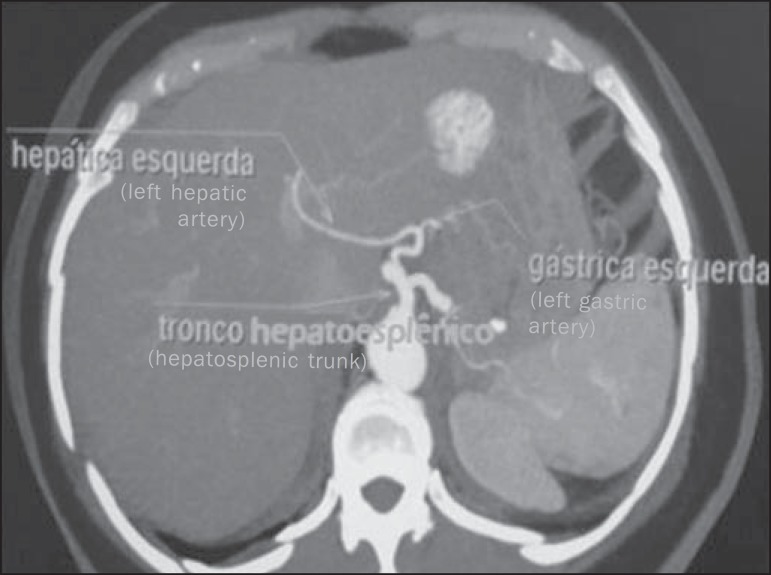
Contrast-enhanced axial computed tomography. The image demonstrates a
